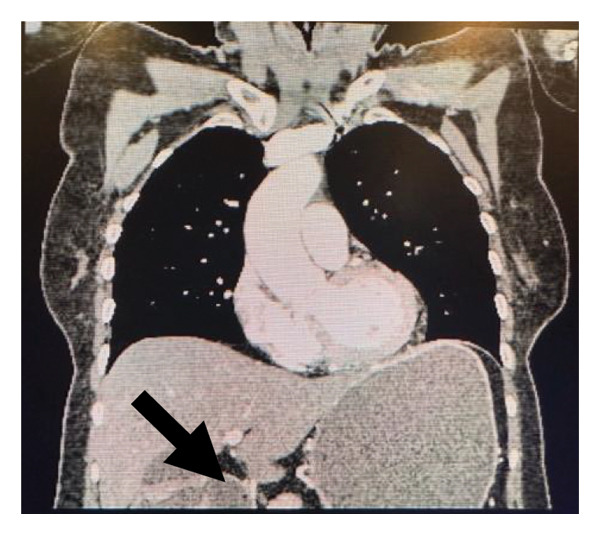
CT coronal view. Arrow showing the stenosis with upstream gastric dilatation.
